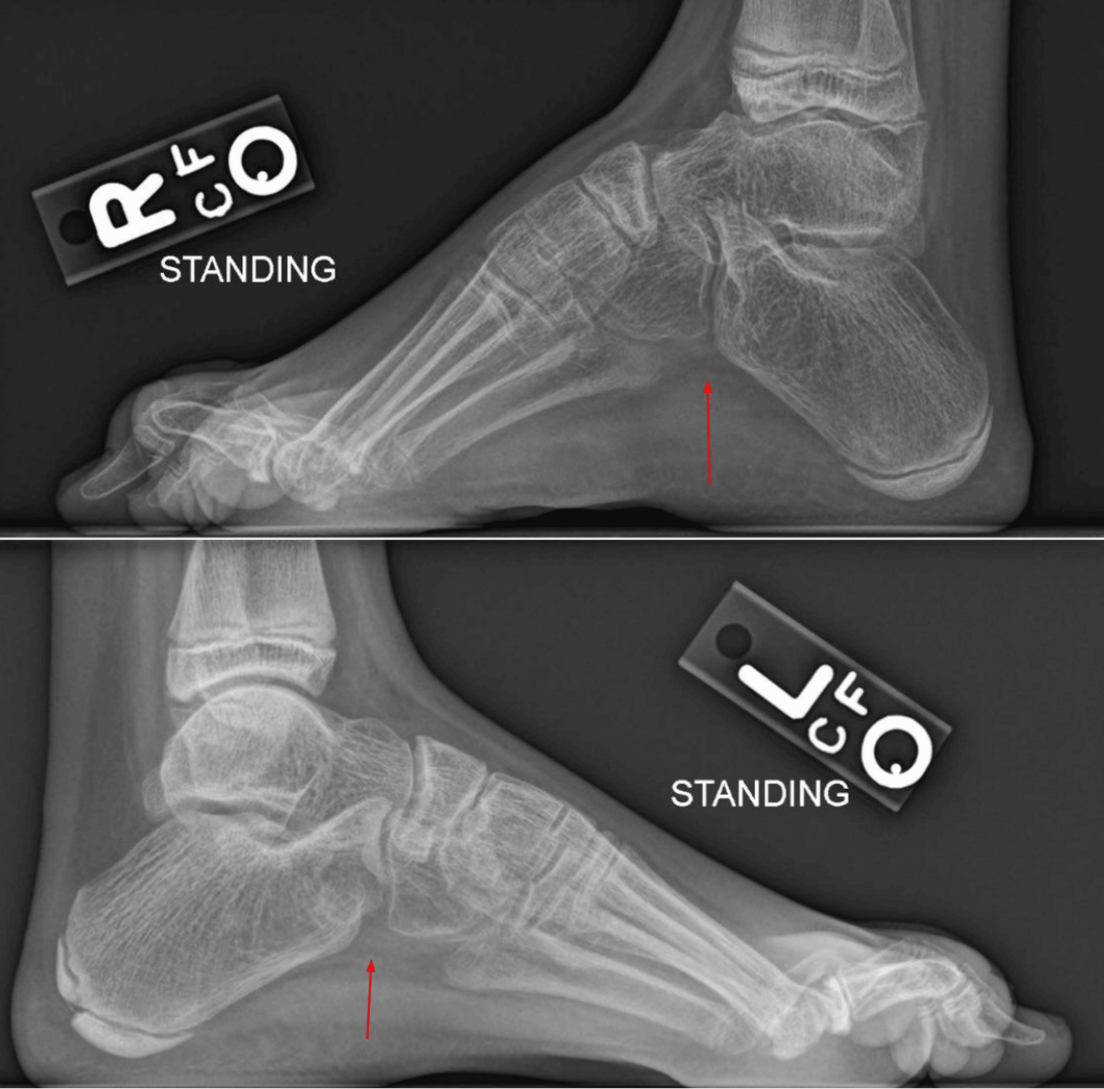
Initial radiographs of bilateral feet show pes cavus, right worse than left, and generalized osteopenia. Red arrows depict bilateral pes cavus.
